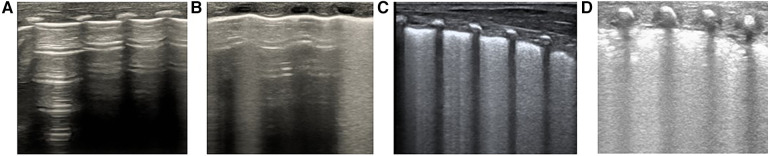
Calculating lung ultrasonography scores. Segmentation: Each lung is divided into 3 regions (anterior L12/R12, lateral L34/R34, and posterior L56/R56), totaling 6 regions. Scoring: The higher the score, the more severe the condition, with a total score of 18 points. The detailed scoring criteria are as follows: (A) 0 points indicate normal lung ventilation, characterized by A-lines with sporadic B-lines; (B) 1 point signifies moderate reduction in lung ventilation, including fused B-lines and interstitial syndrome (B-line accumulation <50% of the lung field examined); (C) 2 points denote severe reduction in lung ventilation, with a small amount of localized subpleural consolidation (B-line accumulation ≥50% of the lung field examined; B-line covers more than half of the intercostal space); (D) 3 points represent extensive lung consolidation.
